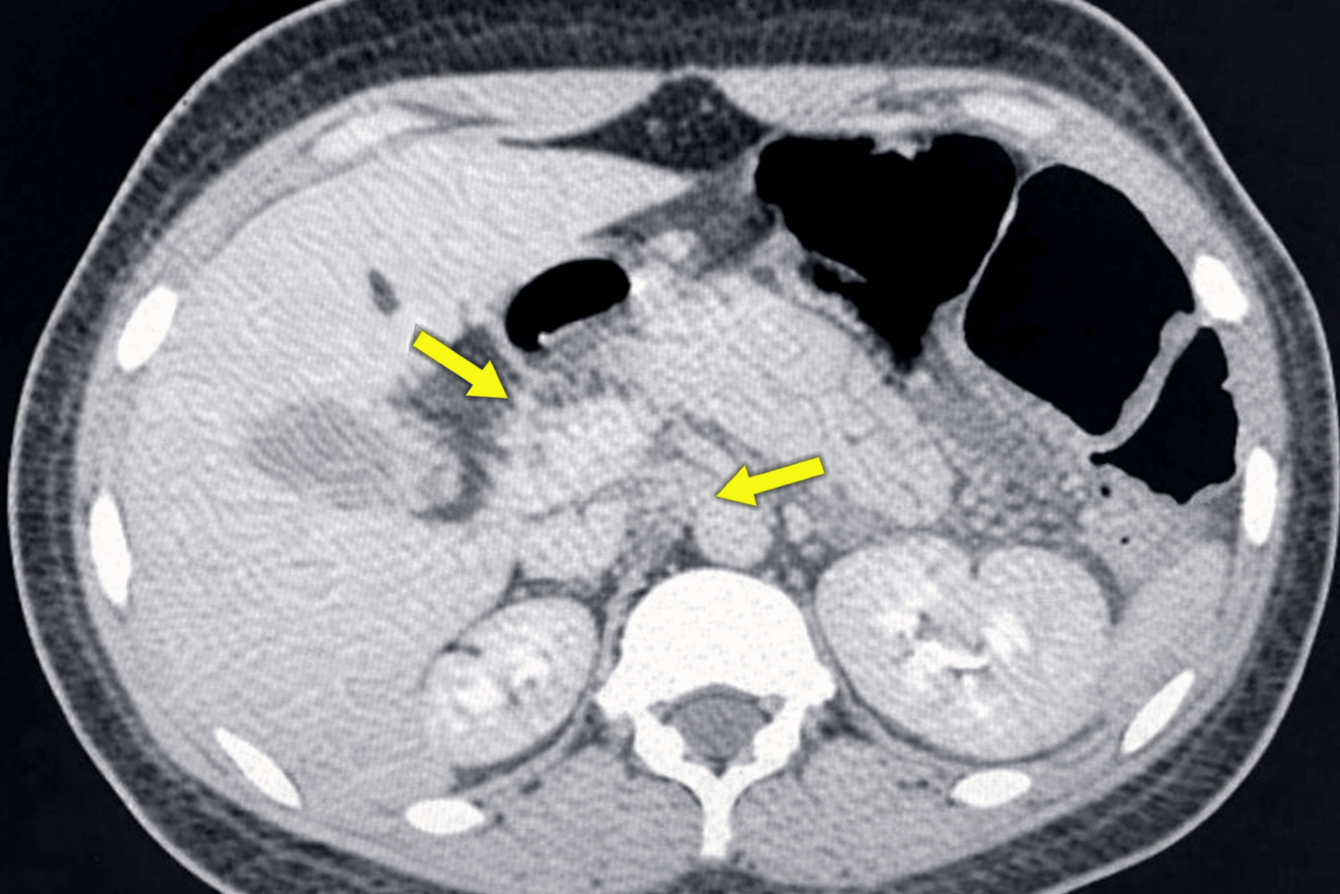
Initial contrast-enhanced computed tomography findings of severe acute pancreatitis Axial contrast-enhanced computed tomography of the abdomen demonstrating diffuse enlargement of the pancreas with ill-defined margins and surrounding peripancreatic fat stranding (yellow arrows), consistent with edematous severe acute pancreatitis at presentation.
